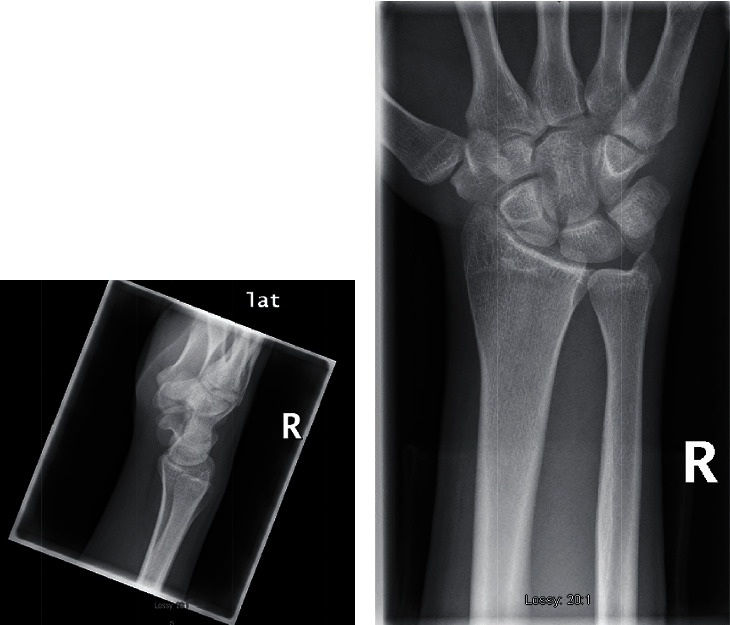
(a, b) X-ray right wrist PA and lateral views suggestive of a dual hamate fracture.
